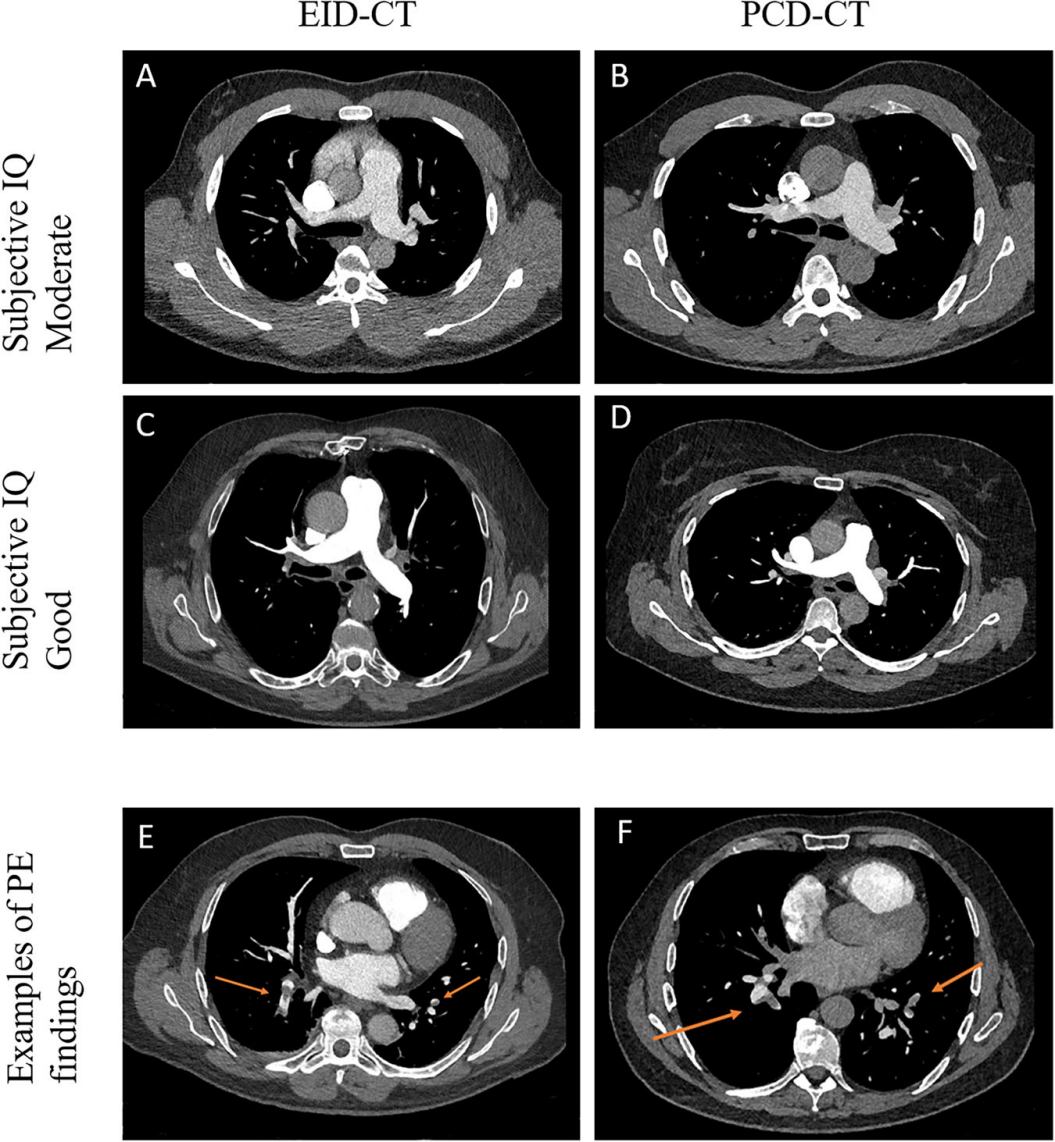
Representative CT pulmonary artery scans from both a PCD-CT and an EID-CT. Images A, B were rated by both observers as moderate, and C, D were rated as good by both observers. Images E, F are examples of PE findings for both scanners
